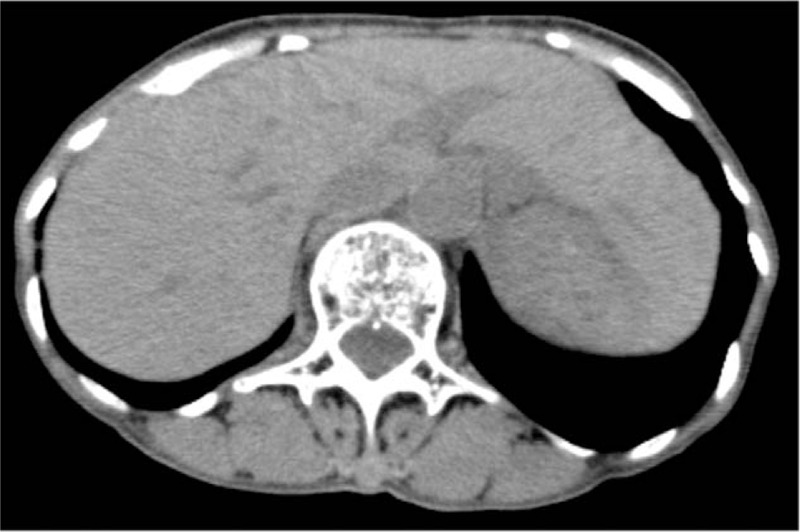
Imaging study of the abdominal computed tomography at hepatitis B virus reactivation.
